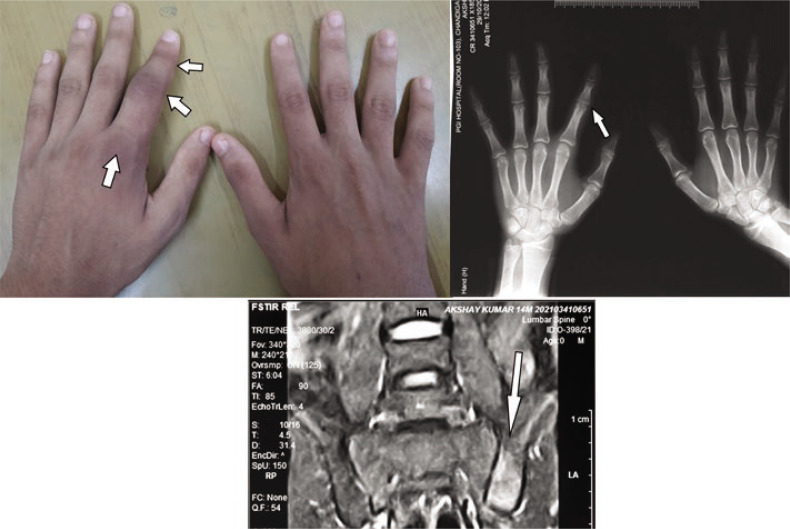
Upper panel (left): Swollen left second MCP, PIP and DIP joint resembling a ‘ray pattern’ of joint involvement. Upper panel (right): Hand radiograph showing reduced joint space of left second PIP joint with small erosions. Lower panel: MRI sacroiliac joint (STIR) showing bone marrow edema suggestive of acute sacroiliitis (left).
